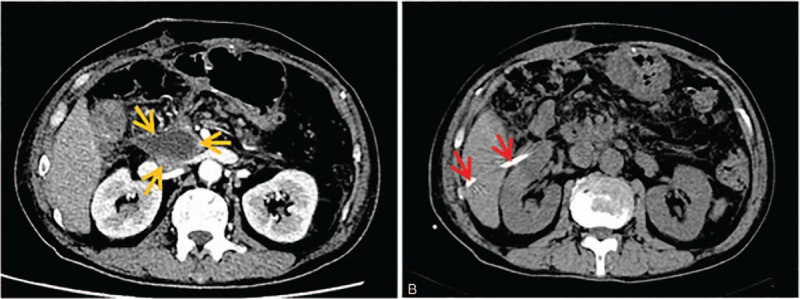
Transhepatic sixth segment approach. (A) Axial CT scans showed that an abscess (light orange arrow) with size of 3 × 4 cm was detected in previous region of pancreatic head and spread to the porta hepatis. (B) Axial CT scans showed drainage catheter (red arrow) get through the sixth segment of liver parenchyma into the abscess cavity and abscess cavity completely collapsed 12 days after the placement of drainage catheter.
